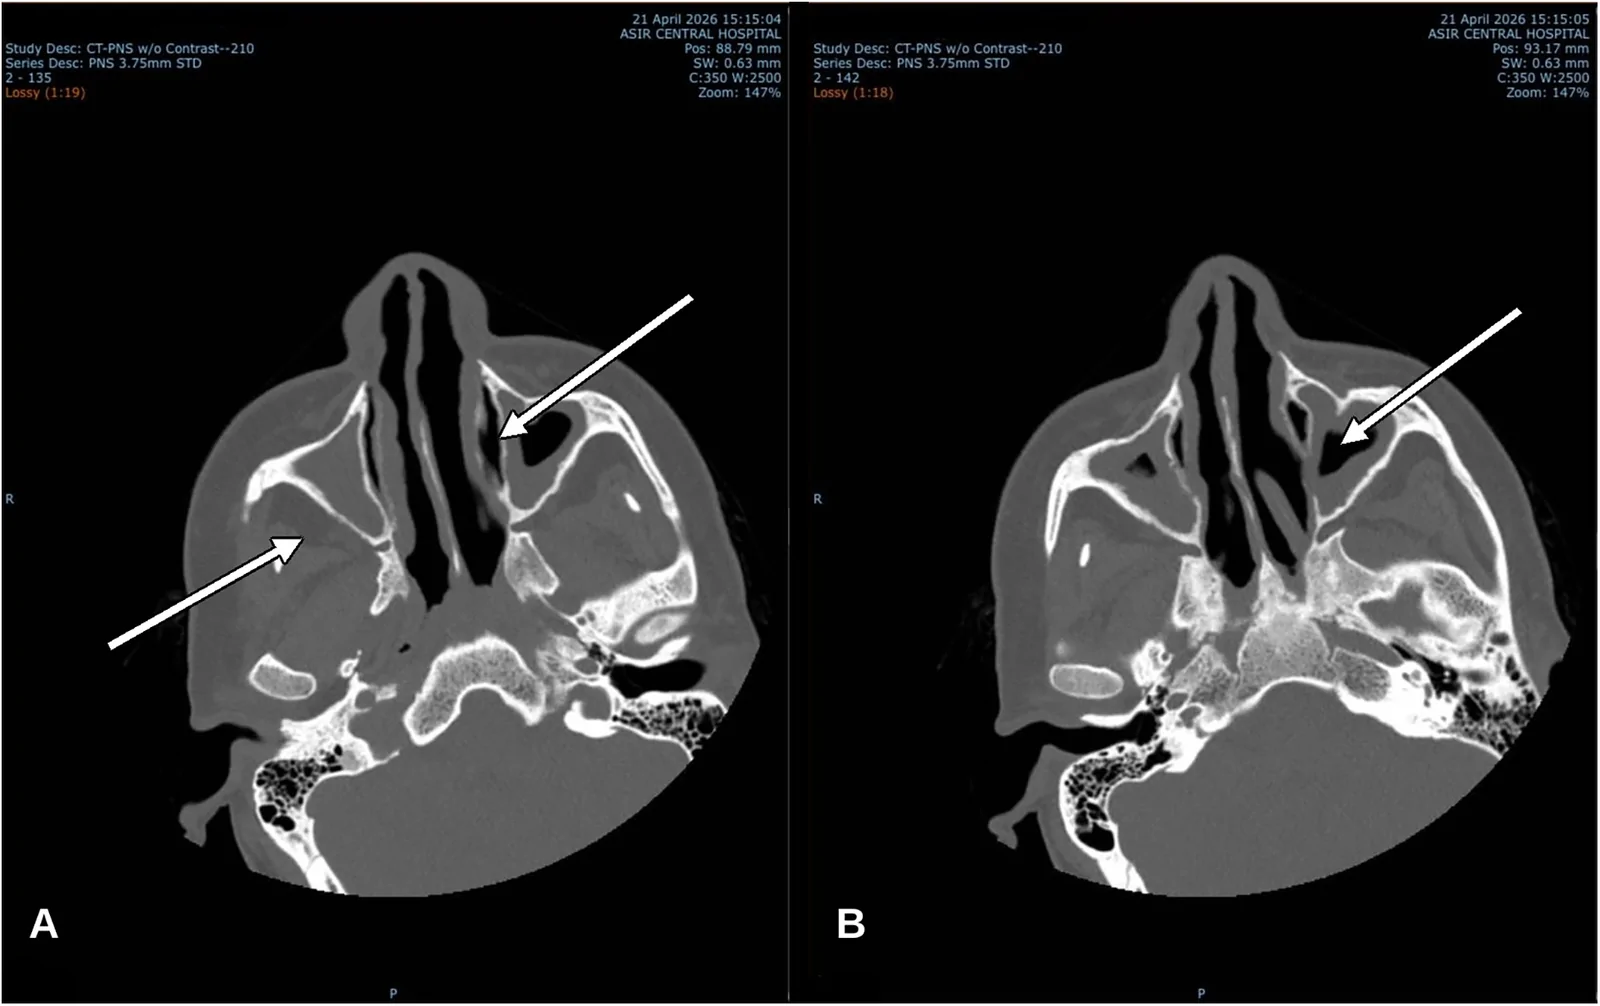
Axial CT of the paranasal sinuses (bone window), 21 April 2026, in the early period after revision FESS, showing post-surgical sinonasal cavities with residual mucosal disease and bony remodeling. (A) Maxillary/nasal level; the upper arrow indicates the widened left maxillary antrostomy and the lower arrow the residual opacification of the right maxillary sinus. (B) Ethmoid-sphenoid level; the arrow indicates the surgically opened left ethmoid cavity. Patient identifiers redacted.
